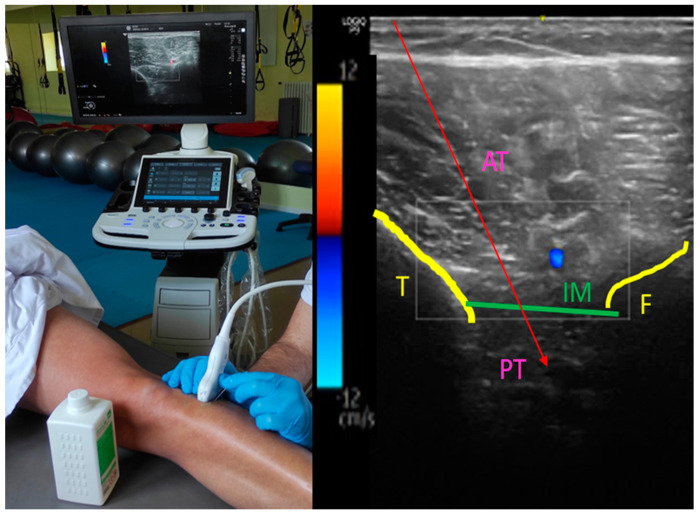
Ultrasound−guided dry needling. Upper third of the leg; AT, followed by the interosseous membrane (IM) as a hyperechoic band between the tibia (T) and fibula (F), sparing the neurovascular component (anterior tibial artery and vein, and deep peroneal nerve), to finally visualise PT.
